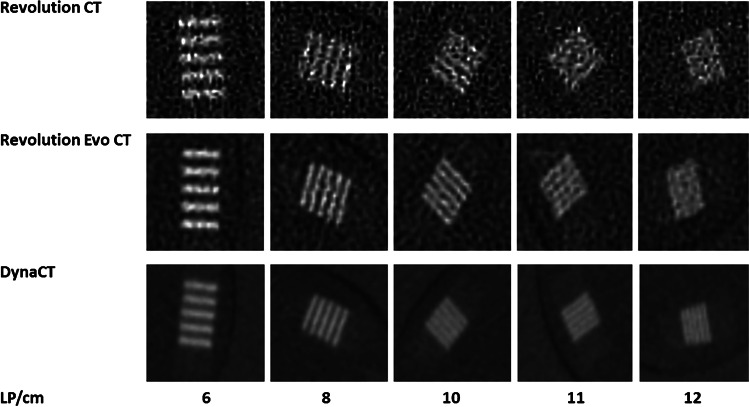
Spatial resolutions of the line pair inserts for the Revolution CT, Revolution Evo CT and the DynaCT, which were assessed by the radiologists. *LP* line pair
